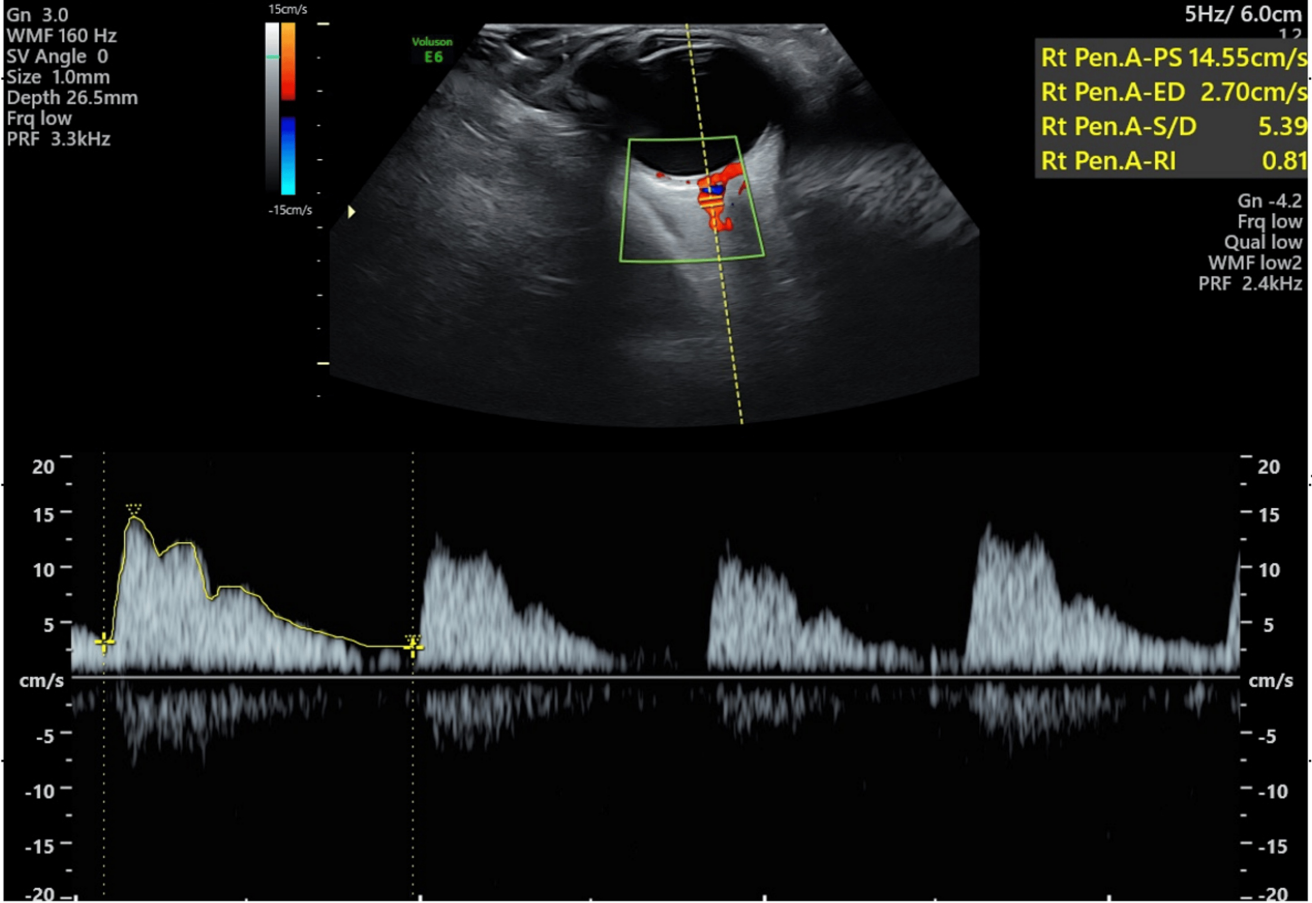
Doppler imaging of the right ophthalmic artery showing mean enveloped velocity and Resistivity Index (RI).
